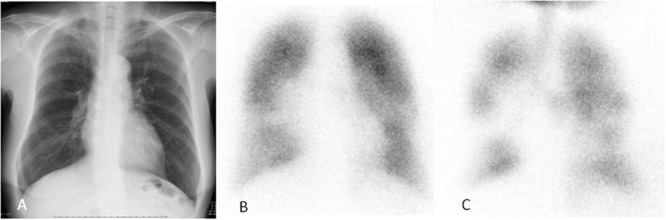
Bronchiolitis obliterans following *M. pneumoniae* infection. (A) Chest X-ray showing normal. (B)
